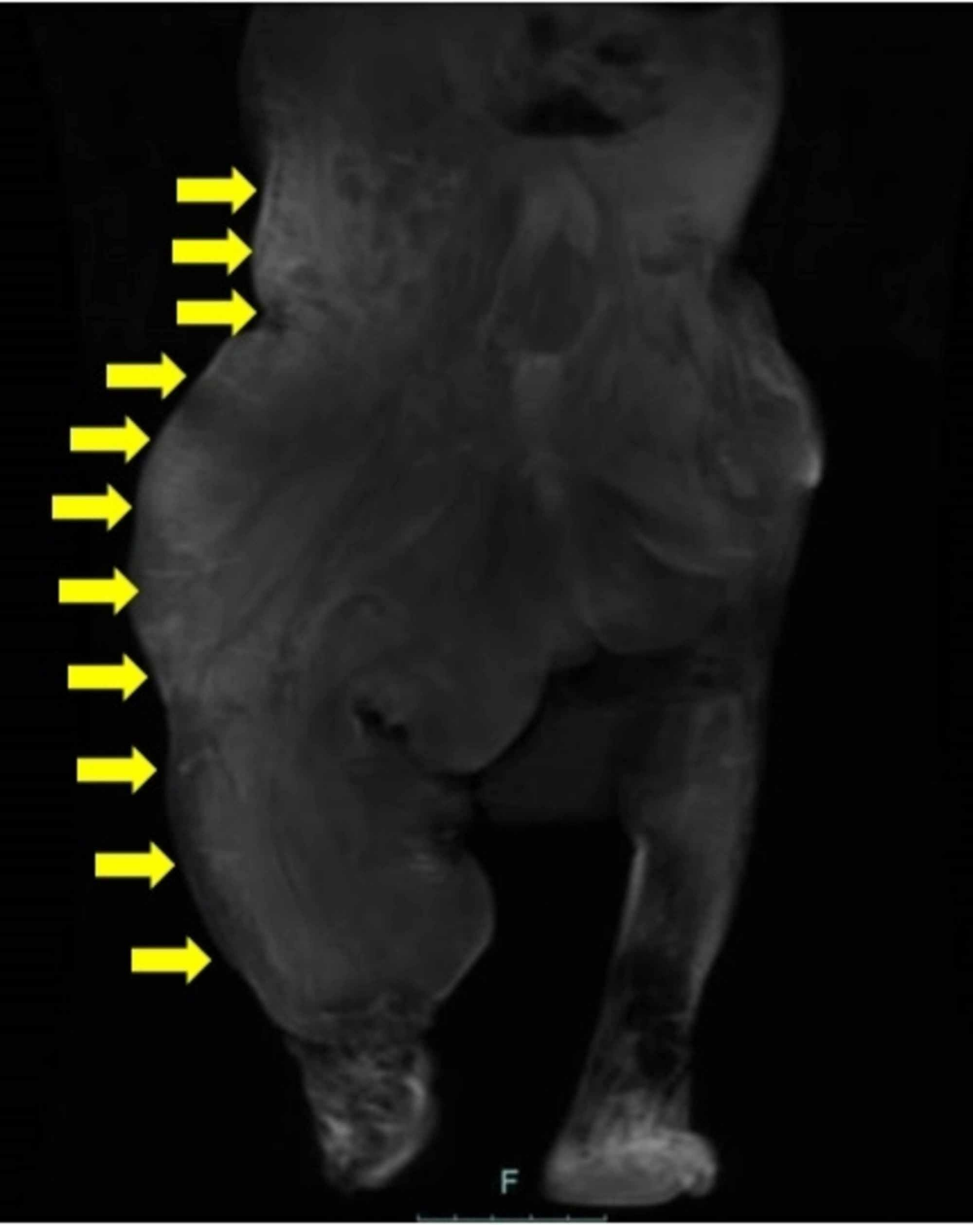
Post-gadolinium T1-weighted coronal magnetic resonance imaging (MRI) scan showed non-enhanced (black) lymphatic malformations in most of the right side of the body (yellow arrows)
